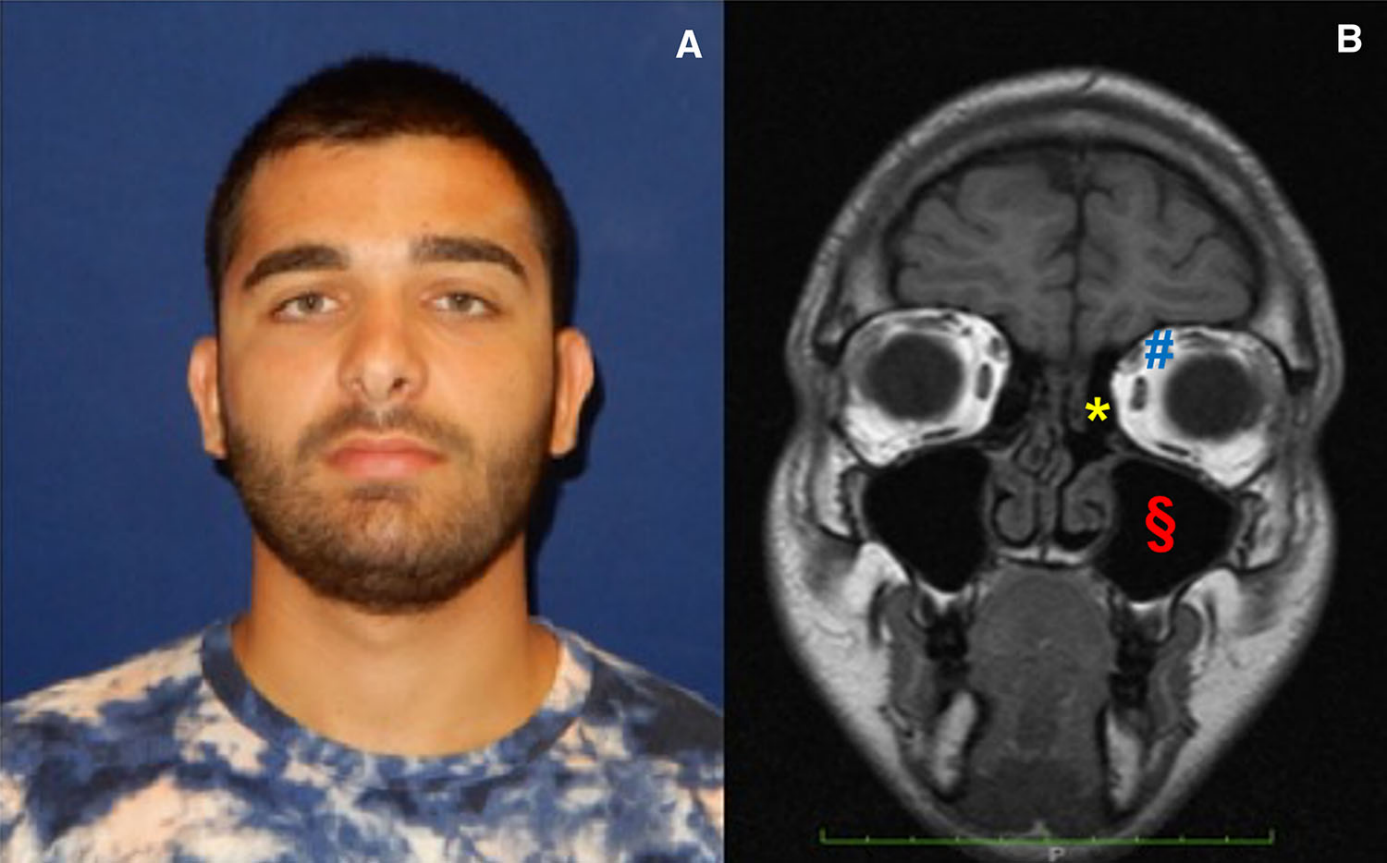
Case 2: Postsurgical photo of patient in frontal section: resolution of swelling induced by abscess in supero-medial section of the left orbit (a) and MRI Imaging in coronal section: maxillary sinus totally empty (§), rehabilitation of periorbital tissues (#), ethmoid site drained of purulent secretions (*) (b)
